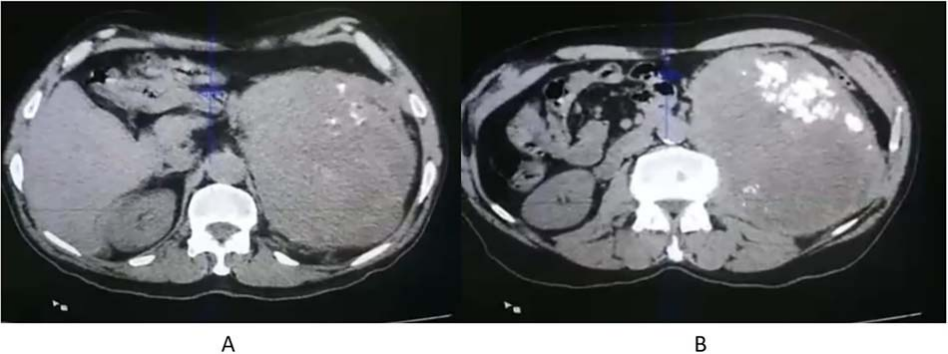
CT scan axial (A, B) showing left renal solid mass, disrupting the upper two-third of renal parenchyma, with thick dystrophic calcifications (B).
